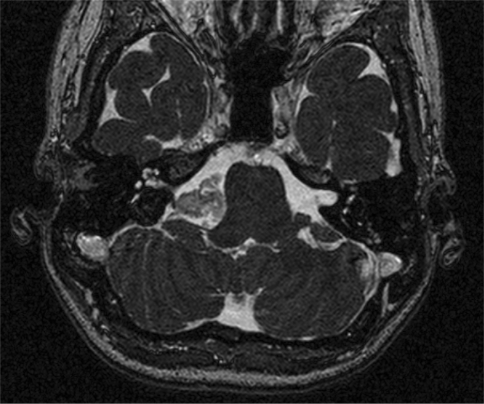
Brain MRI in axial plane and T2 sequences: extraaxial lesion at the right cerebellopontine angle, heterogeneous, hyperintense with edges well defined. The lesion produces imprint on the brain stem without signs of infiltration. All these features are suggestive of an epidermoid cyst
